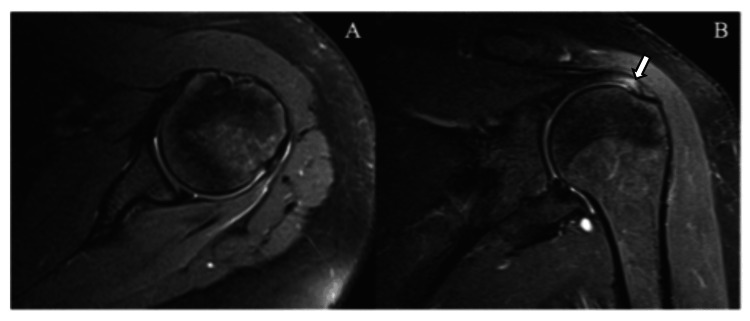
MRI of the left shoulder showed mild rotator cuff tendinosis and the absence of a tear. (A) Axial MRI. (B) Coronal MRI with an arrow pointing to tendinopathy. MRI: magnetic resonance imaging
